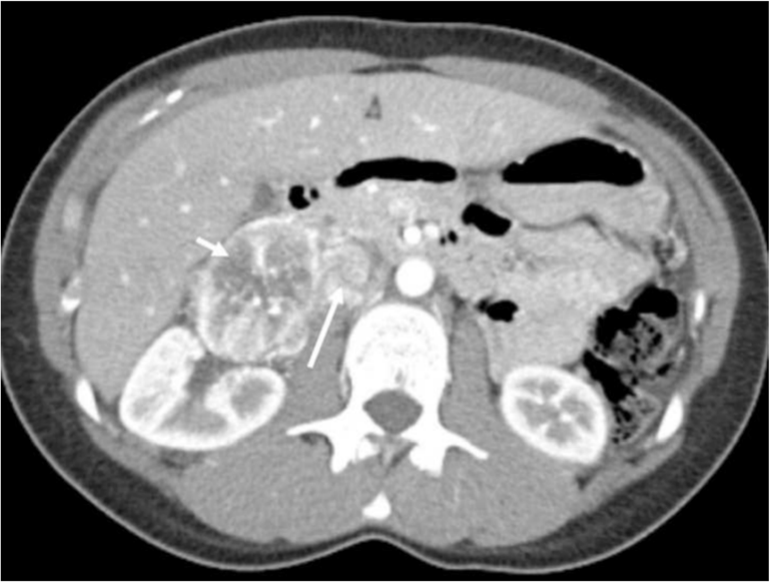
Axial portal venous CT shows a heterogeneous mass (*short arrow*) at the right renal hilum, with invasion of the inferior vena cava (*long arrow*). This was excised and was pathologically shown to be a phaeochromocytoma
